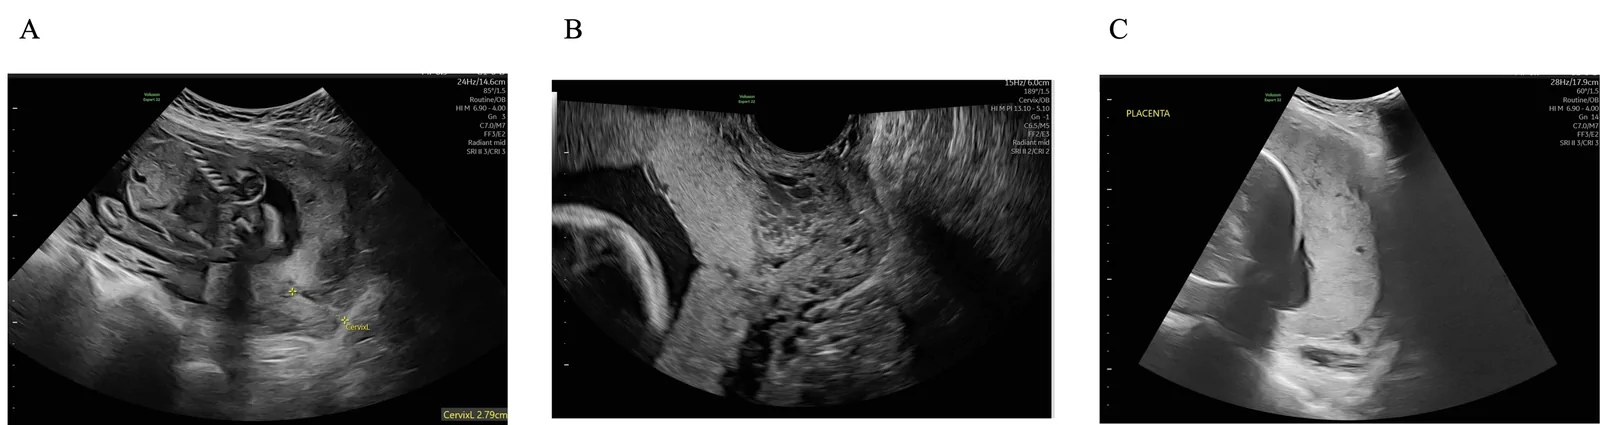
Prenatal Ultrasound Findings Demonstrating Persistent Complete Placenta Previa Without Sonographic Evidence of Placenta Accreta Spectrum (A) Transabdominal ultrasound obtained at 20 weeks demonstrating complete placenta previa without sonographic evidence of placenta accreta spectrum. The hypoechoic retroplacental space is clearly delineated, and the retroplacental myometrium is of normal thickness.
